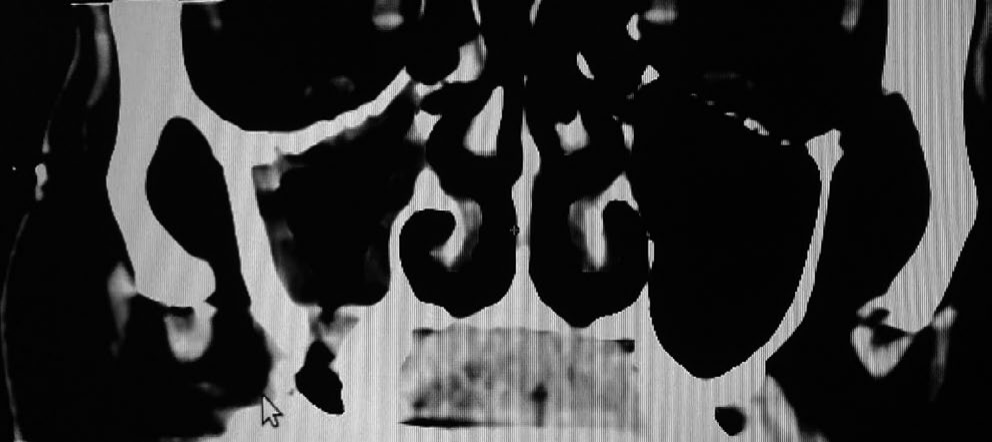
Coronal section CT scan reveals bone discontinuity in sinus floor
